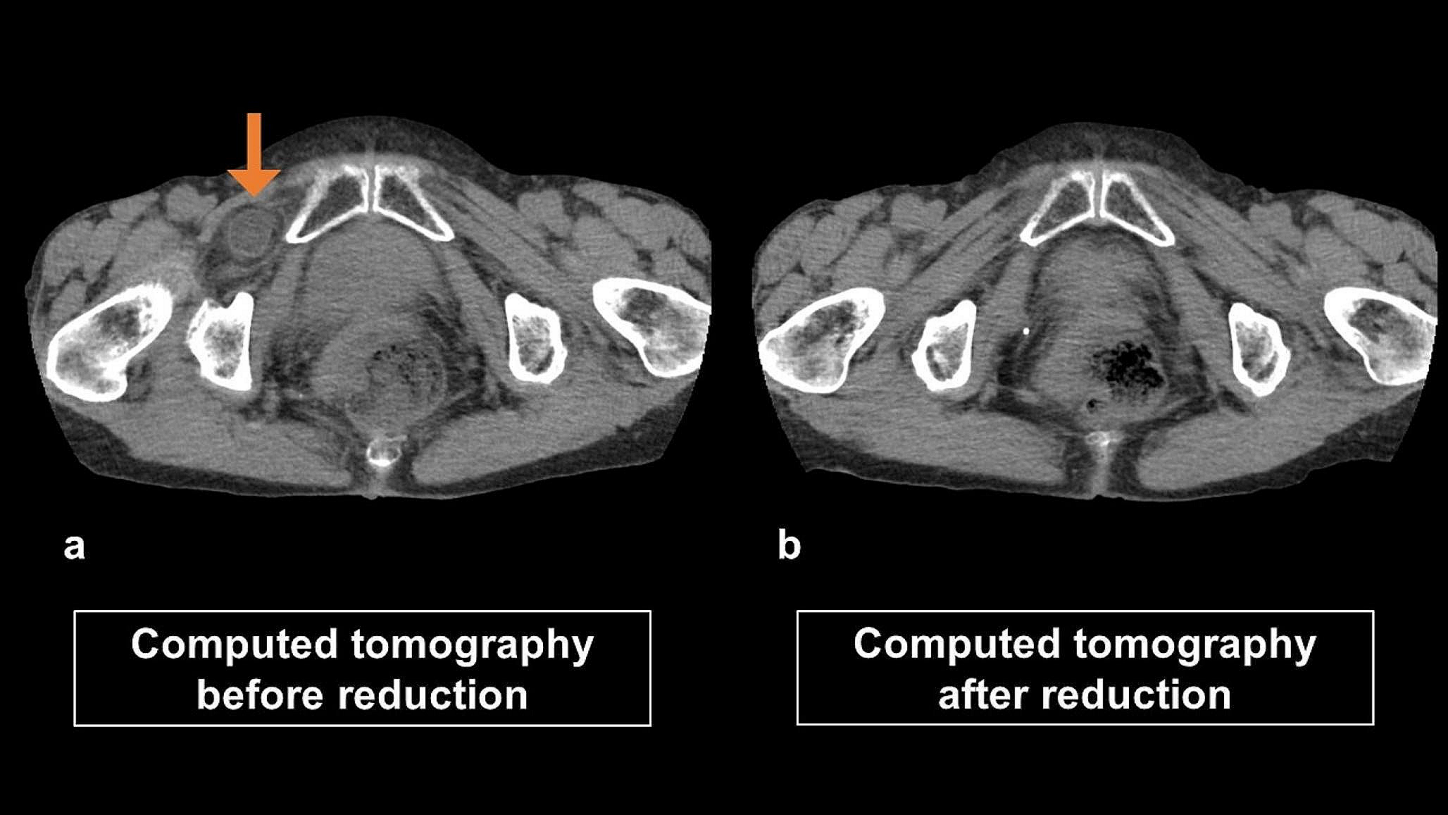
The computed tomography scan images before and after non-invasive reduction
